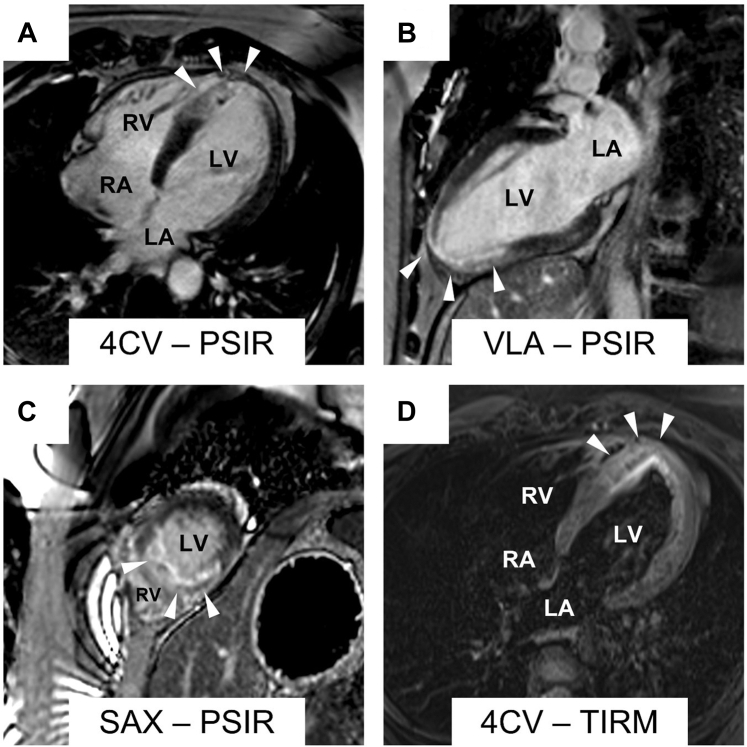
Signs of Ischemic Injury in Cardiac Magnetic Resonance Cardiac magnetic resonance imaging showed signs of subacute myocardial infarction in the territory of the left anterior descending artery. The phase-sensitive inversion-recovery (PSIR) gradient echo sequences (A: four-chamber view [4CV], B: vertical long axis [VLA], C: short axis [SAX]) show late gadolinium enhancement in the apical to midventricular septal region. Turbo-inversion-recovery-magnitude (TIRM) sequences show apical septal and anterior signal enhancement (D). The white arrowheads show signs of subacute myocardial infarction; A to C shows LGE, D shows t2 signal enhancement. LA = left atrium; LV = left ventricle; RA = right atrium; RV = right ventricle.
